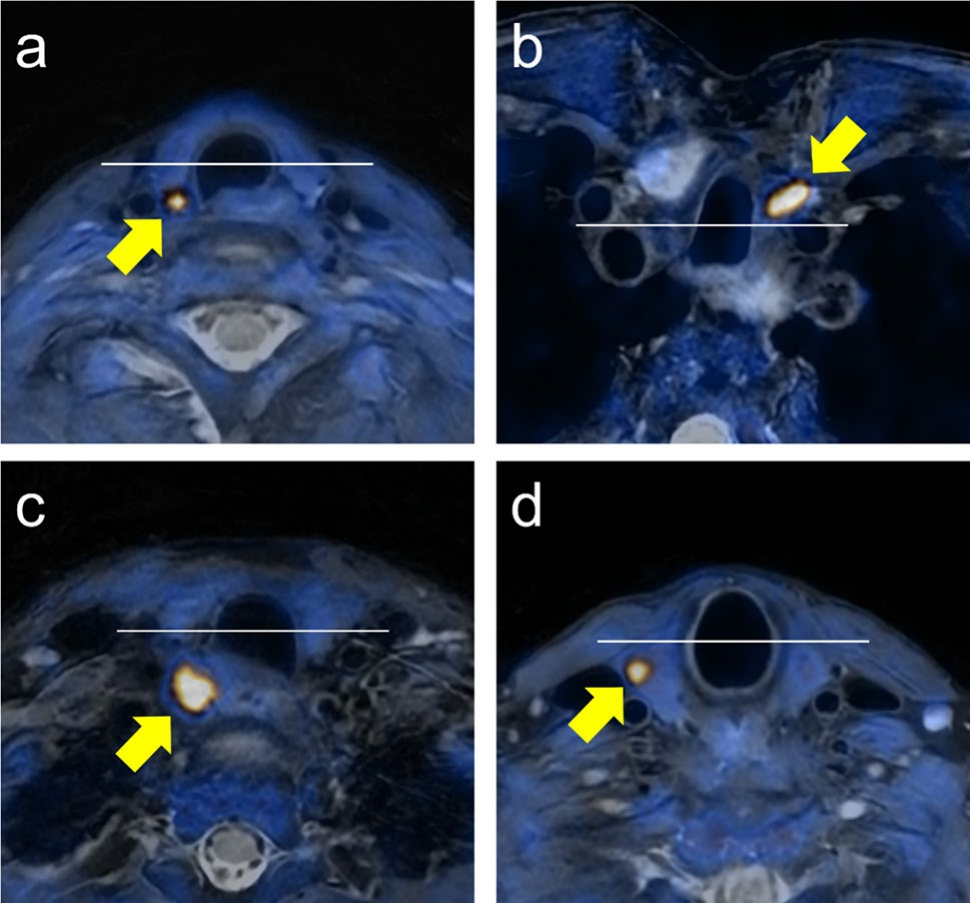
Representative T2-weighted fat-suppressed [^18^F]Fluorocholine PET/MR images of parathyroid adenoma location in relation to the TBL in four different subjects. a Orthotopic upper pole parathyroid adenoma located posterior to the TBL. b Orthotopic lower pole parathyroid adenoma located anterior to the TBL. c Overly descended upper pole parathyroid adenoma located posterior to the TBL. d Subcapsular intrathyroidal upper pole parathyroid adenoma located posterior to the TBL. White line: tracheal bisecting line (TBL); yellow arrows indicate parathyroid adenomas
